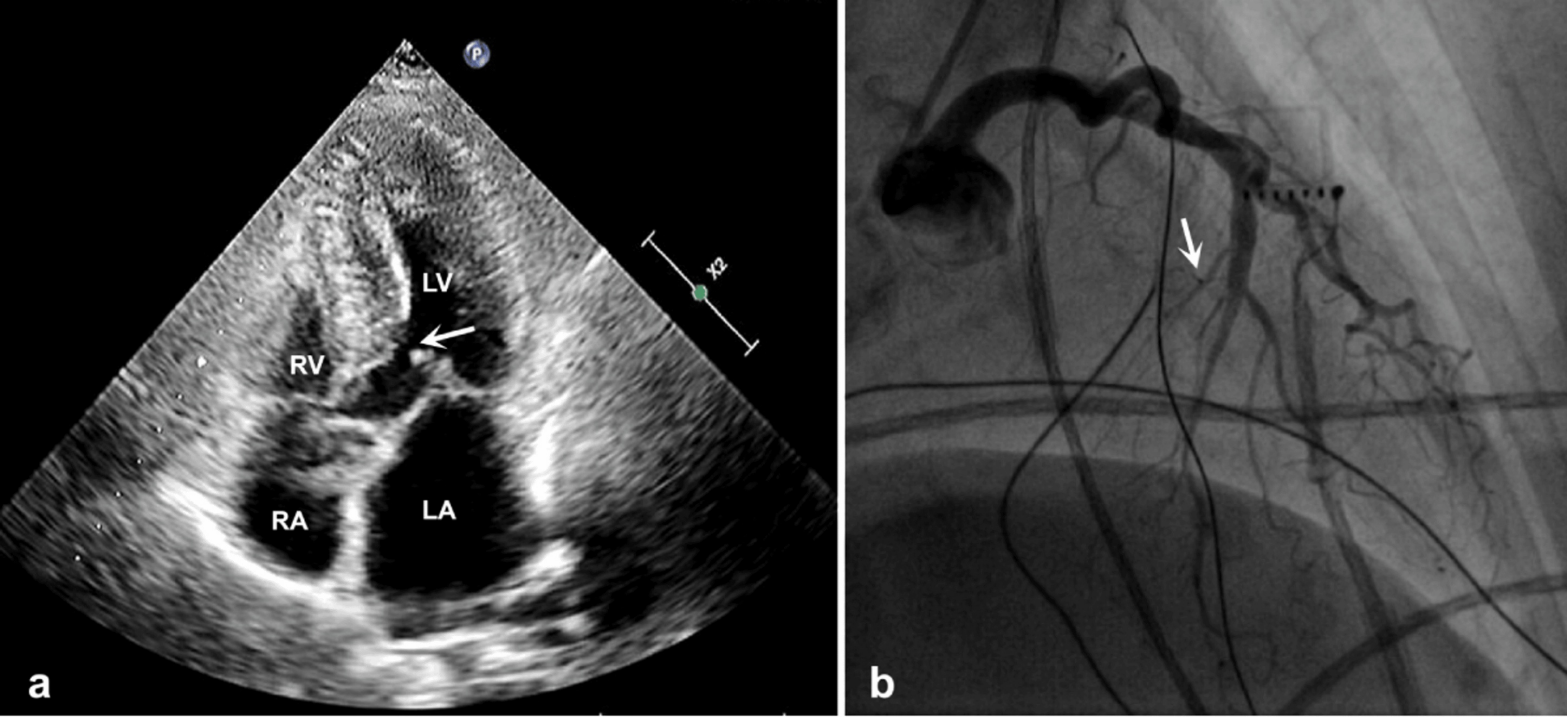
TTE and left coronary angiography before ablation a) TTE shows septal thickening and SAM (white arrow) of the anterior mitral valve leaflet in the apical four-chamber view, in systole. b) Moderate stenosis and small septal branches (white arrow) are seen in the mid-portion of the anterior descending branch. TTE, transthoracic echocardiography; SAM, systolic anterior motion; LV, left ventricle; RV, right ventricle; LA, left atrium; RA, right atrium
